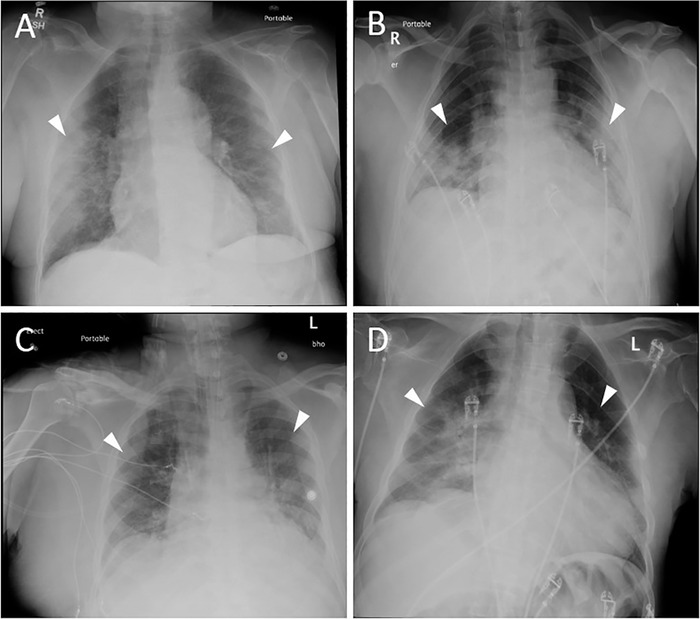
Chest x-ray for (A) 86-year-old mother 2 days after admission shows bilateral ground-glass opacities (white arrowheads), more severe on the right. Chest x-rays for (B) the 58-year-old son, (C) the 71-year-old son, and (D) the 61-year-old son all displayed bilateral airspace opacities on emergency department presentation (white arrowheads).
